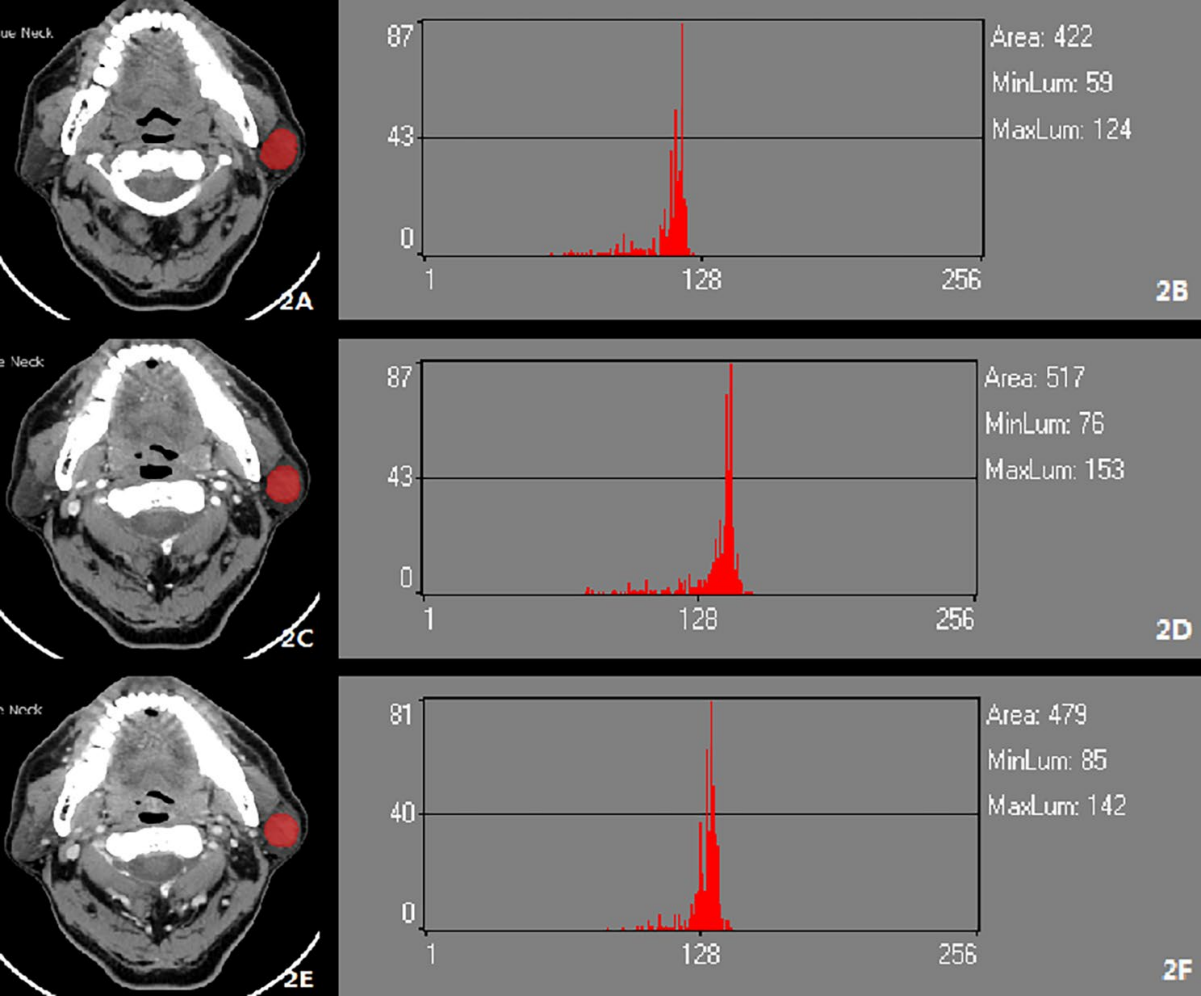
Left pleomorphic adenoma (A) ROI in FSP of Enhanced CT; (B) The histogram of ROI in FSP of Enhanced CT; (C) ROI in AP of Enhanced CT; (D) The histogram of ROI in AP of Enhanced CT; (E) ROI in P of Enhanced CT ; (F) The histogram of ROI in VP of Enhanced CT
